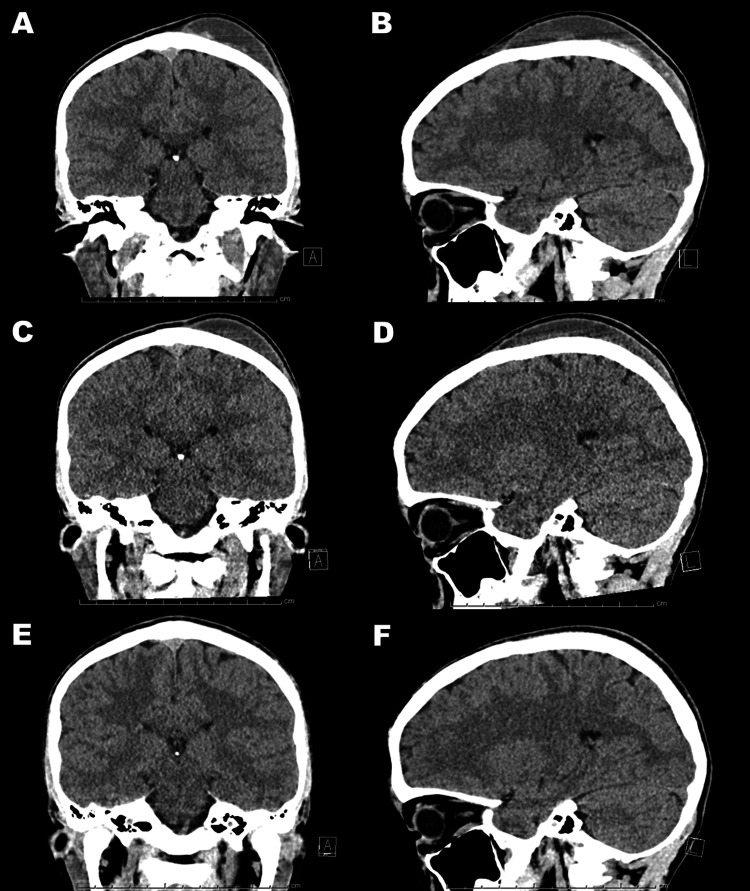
Follow-up CT scan images. The C-SPOH (A, B), through the reaccumulating (C, D), shrinks to provide the cure, which is demonstrated on follow-up CT scan of Day 57 (E, F). C-SPOH, calvarium subperiosteal hematoma
